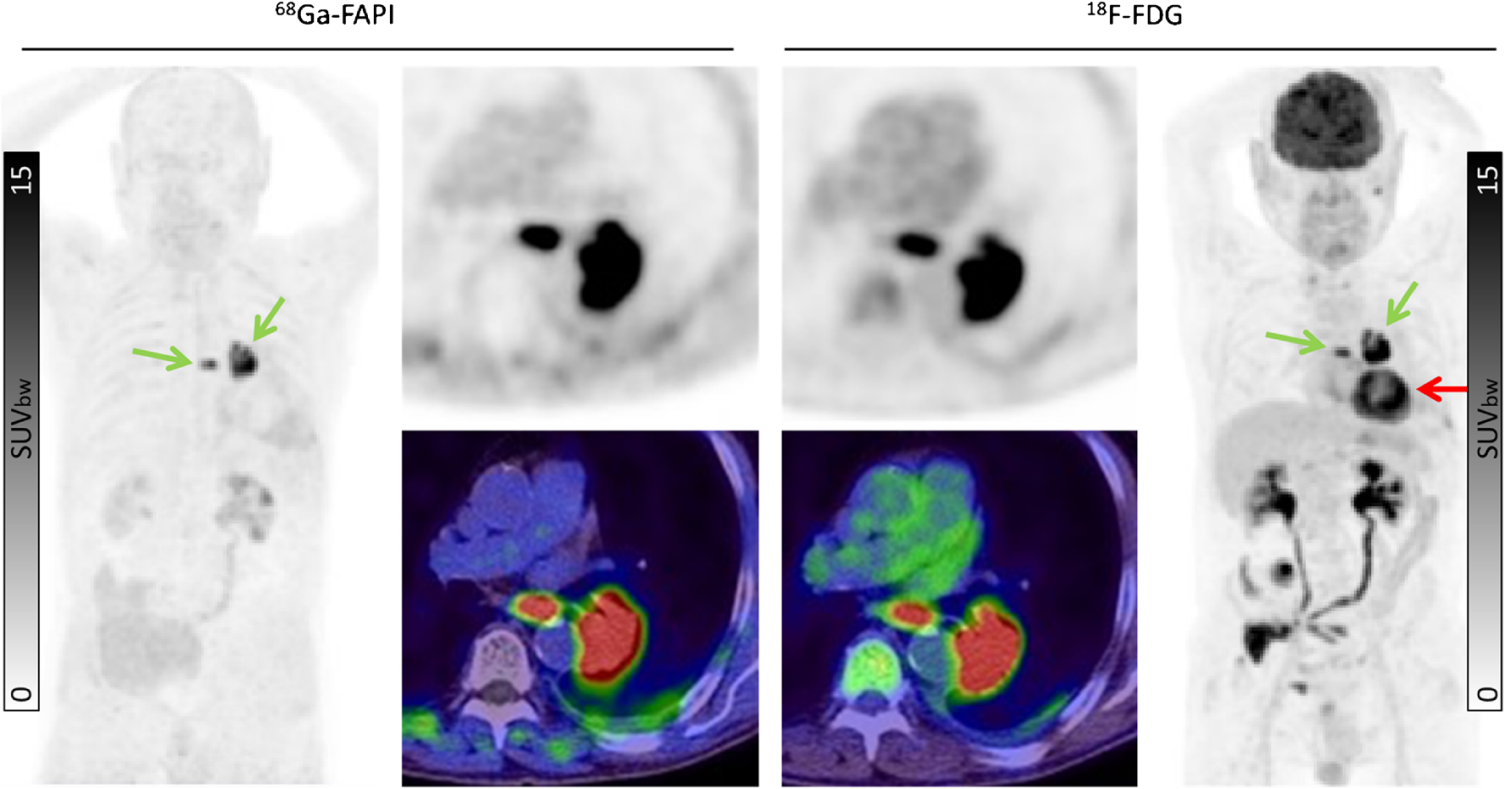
An 80-year-old male patient with lung cancer (green arrows) was diagnosed by ^18^F-FDG PET/CT. ^68^Ga-FAPI PET/CT demonstrated similar tracer uptake (SUVmax: ^18^F-FDG 15.99 vs. ^68^Ga-FAPI 17.95). One advantage of ^68^Ga-FAPI in this instance is the lack of cardiac muscle uptake which is prominent with ^18^F-FDG (red arrow)
